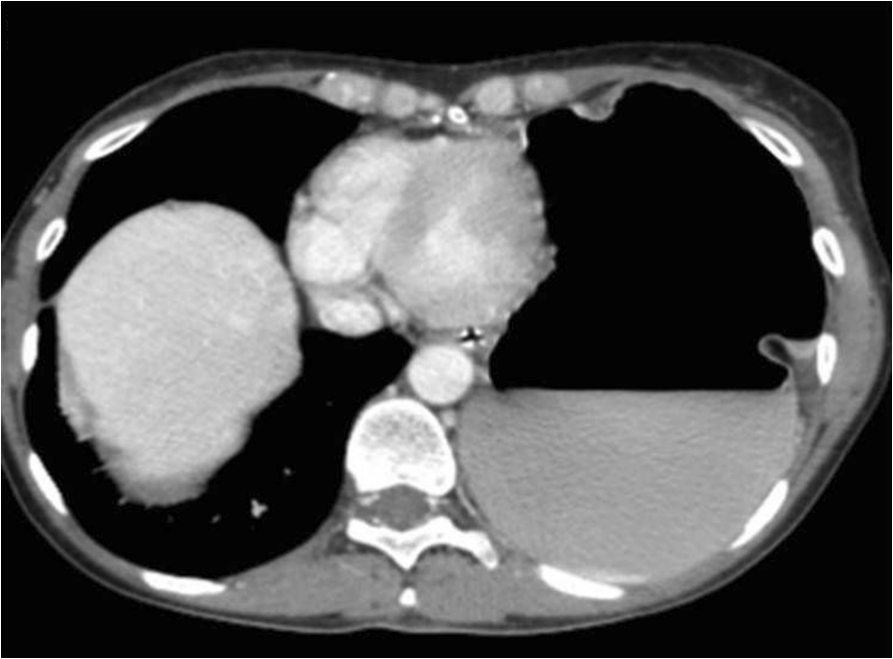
Preoperative plain computed tomographic scan showing left hemidiaphragm lifting and gastric overdistension.
